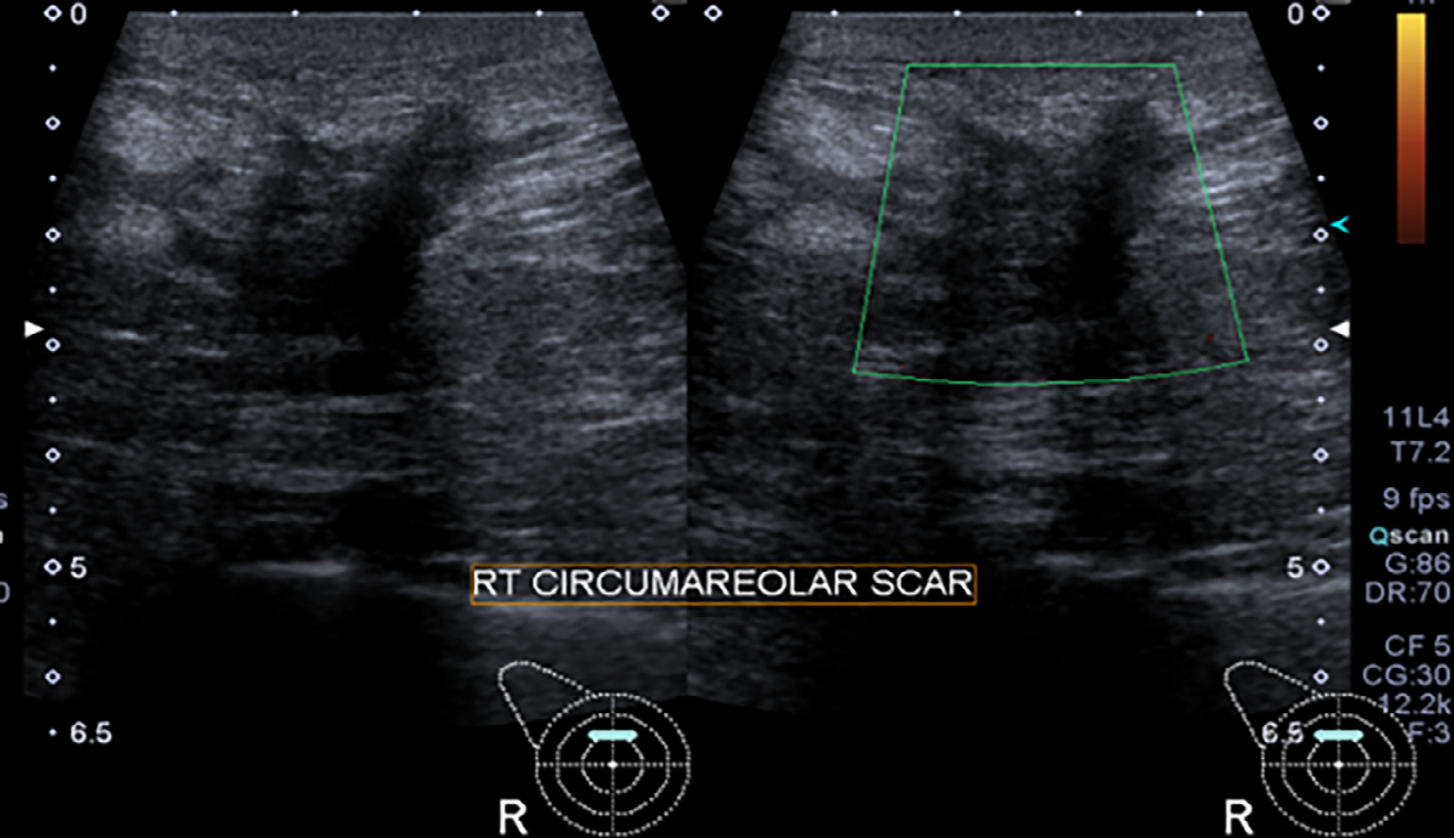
First postoperative ultrasound (performed adjunct to the mammogram) shows a mildly thickened circum-areolar scar with no suspicious nodularity or abnormal vascularity on color Doppler. Biopsy of the scar shows no evidence of recurrence.
